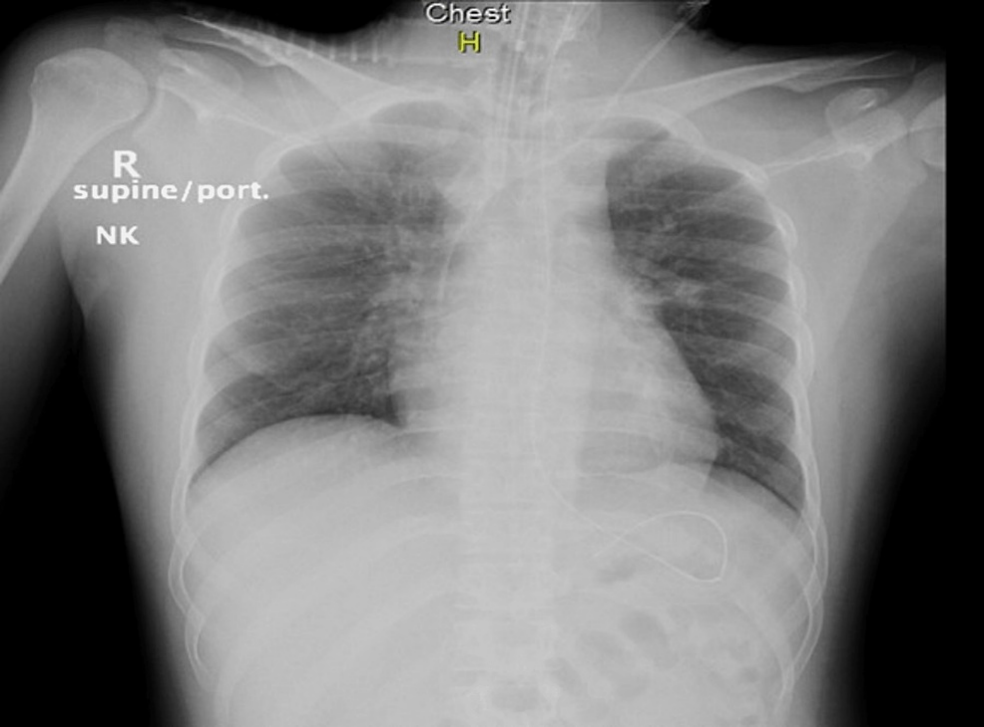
Chest radiograph on admission A nasogastric tube, an endotracheal tube, and a central venous line are seen on the radiograph.
